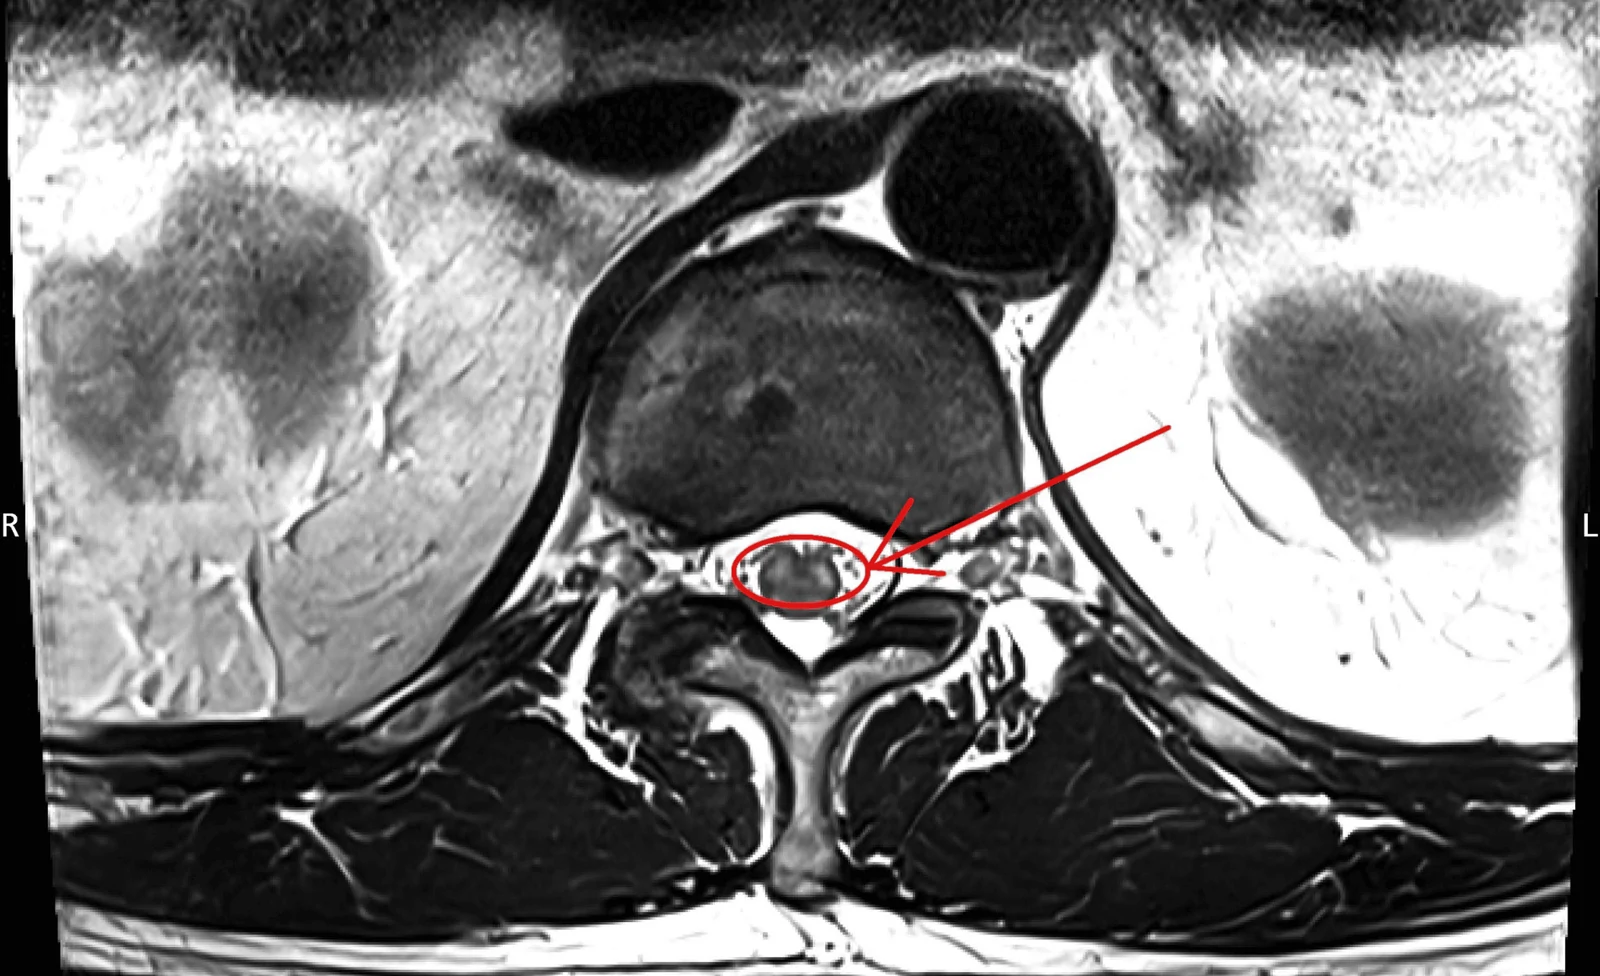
Axial T2-weighted MRI of the thoracic/lumbar spine Axial T2-weighted MRI image at the T12/L1 level demonstrates a focal intramedullary hyperintense signal abnormality involving the anterior gray matter/anterior horn region, indicated by an arrow. T2-weighted images specifically highlight water and fluids to find abnormalities like swelling or inflammation in comparison to an MRI. This finding is supportive of anterior horn cell involvement in the setting of suspected West Nile virus–associated acute flaccid myelitis.
